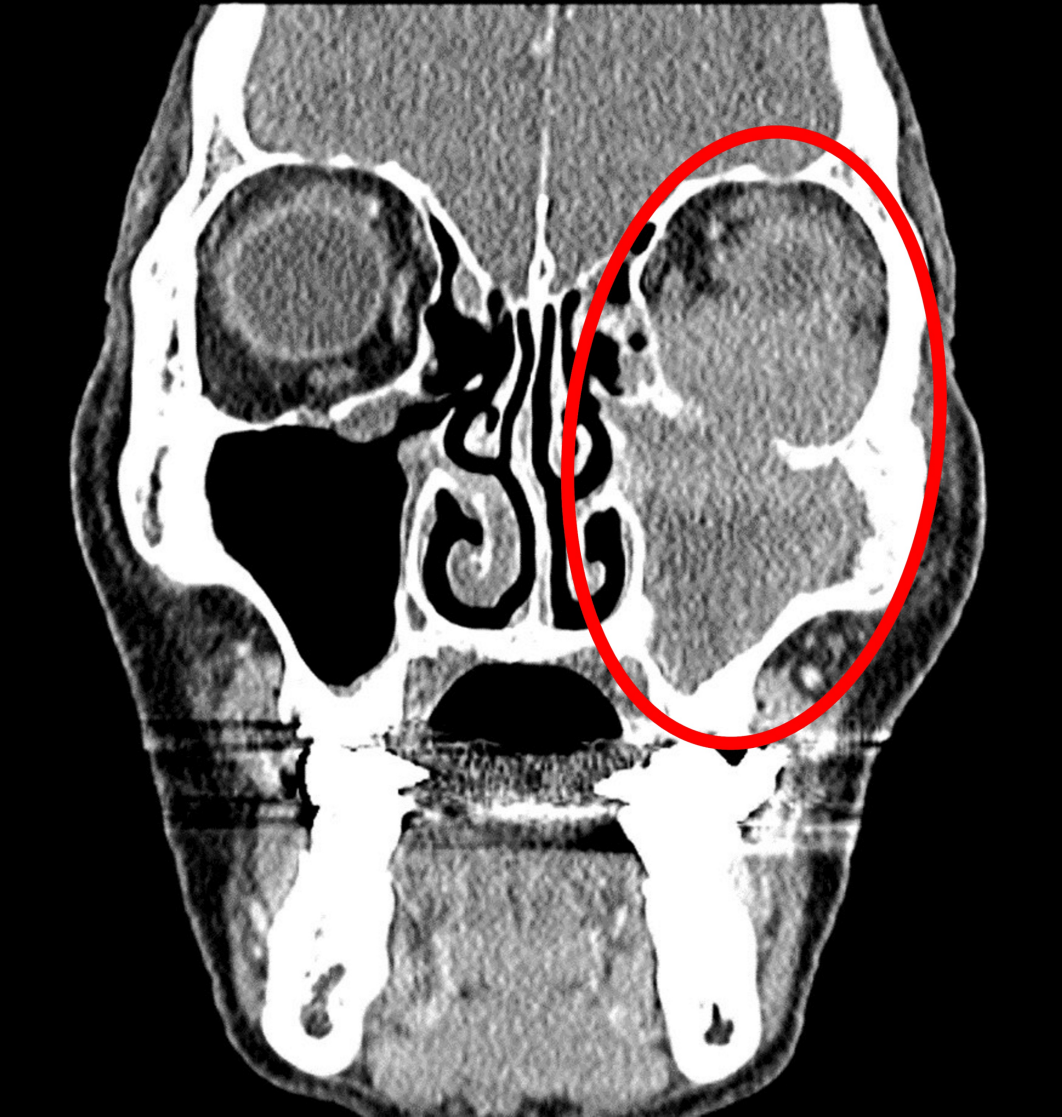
Contrast-enhanced CT image Occupying lesion centered on the left maxillary sinus, with uniform mild contrast in the left orbit and buccal subcutis (circle area). CT, computed tomography
